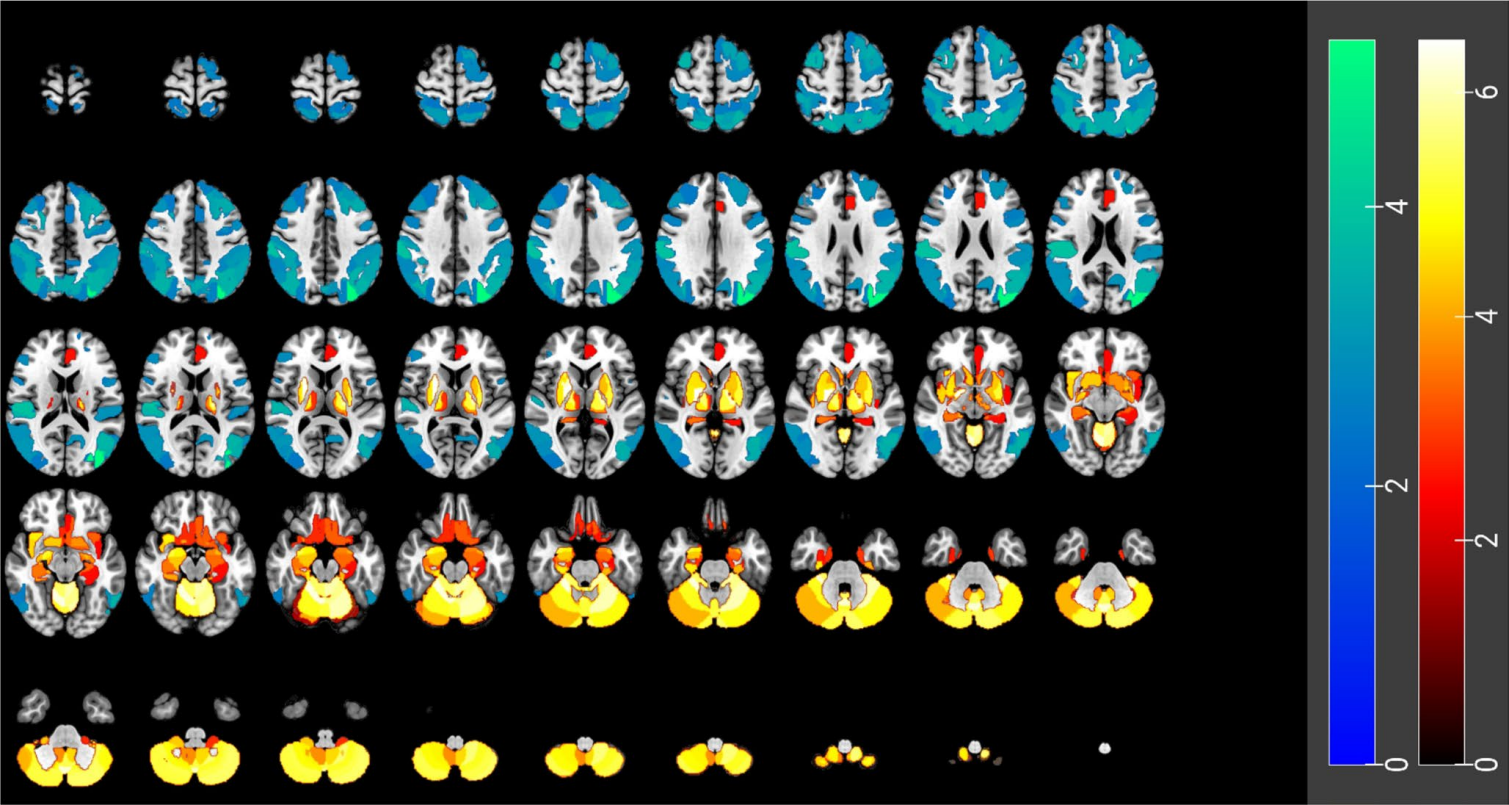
Statistical results: Statistical region-based comparison showing a significant difference in metabolism (FDR corrected *p* < 0.05), highlighting areas where cluster 2 differed significantly from cluster 1. Cluster 2 showed higher metabolism in bilateral basal ganglia, thalami, medial temporal lobes, amygdalae, hippocampi, insular cortices and cerebellum than cluster 1 and lower metabolism in widespread cortical regions including frontal, parietal, occipital and lateral temporal. Increases (red/yellow, “4 hot” colour scale) and decreases (blue/turquoise, “5winter” colour scale), overlaid on MRI template (MNI152) in MRIcroGL [17]. The colour scale represents t-values. Abbreviation: FDR = false discovery rate
